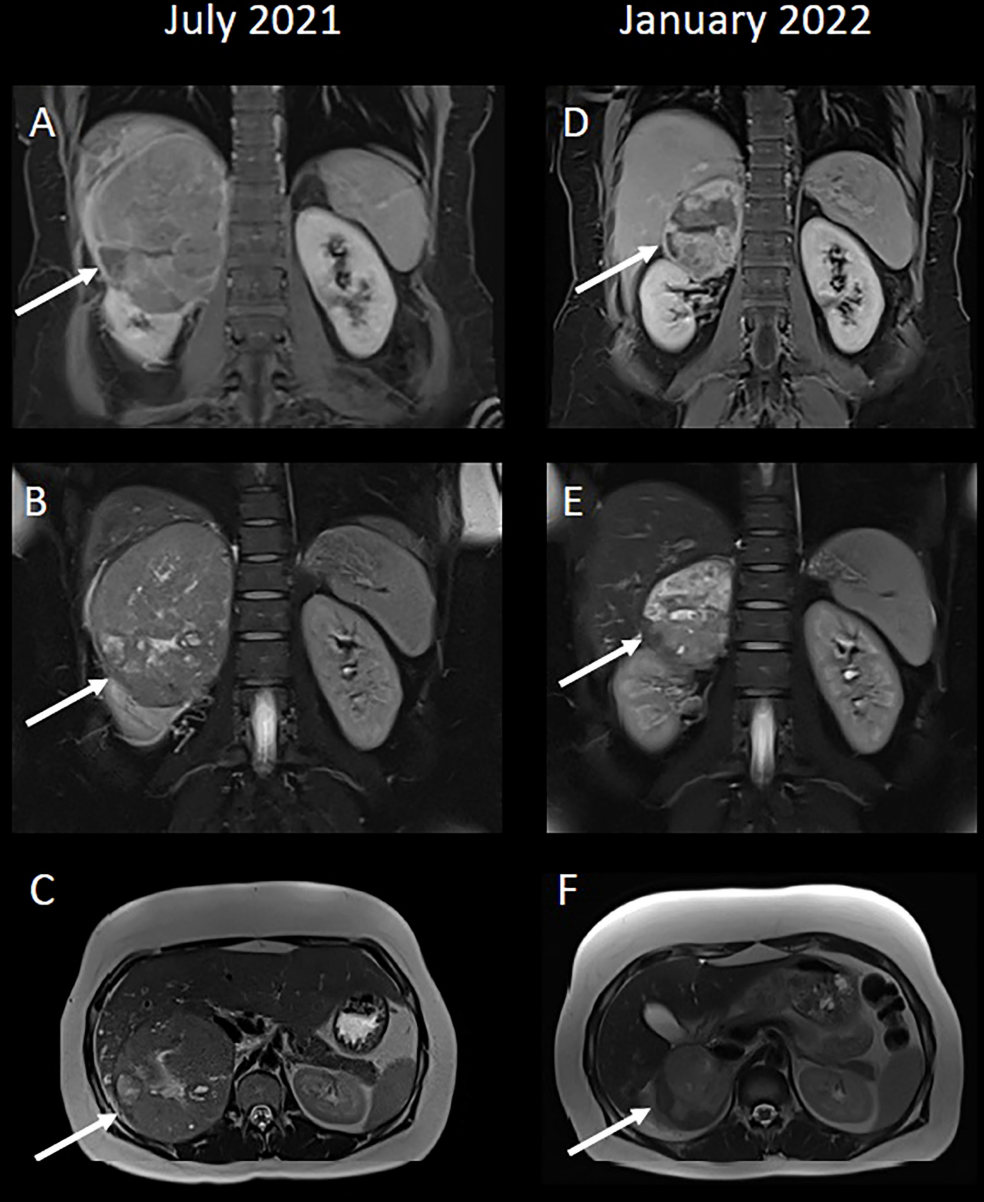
(A, D) Coronal T1-weighted VIBE-Dixon images, 5 minutes post-contrast. (B, E) Coronal T2-weighted HASTE fat-saturated images. (C, F) Axial T2-weighted HASTE images. (A–C) A large mixed signal mass, again suspected to arise from the upper pole of the right kidney. 2A demonstrates contrast-enhancement within the liver parenchyma adjacent to the mass, which was initially suspected to represent hepatic parenchymal invasion. (D–F) A significant reduction in size to the mass post-treatment. Following systemic therapy there is the impression of a fat plane between the right kidney and the mass itself, particularly in 2D, such that the possibility of this mass arising from the adrenal gland, rather than kidney, could have been highlighted.
